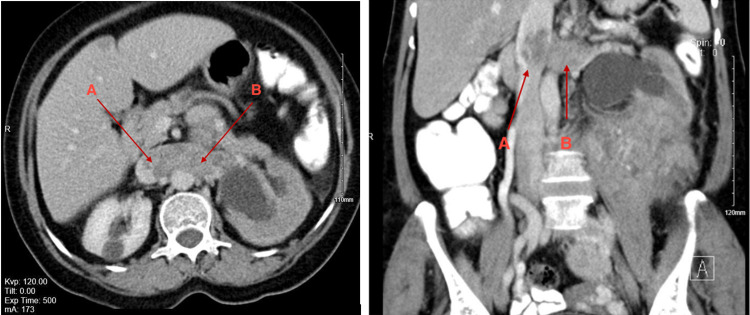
CT scan with contrast showed involvement of IVC and Left Renal Vein as pointed with the arrows. In both left-hand (axial view) and right-hand side (coronal) CT scan images- A: leiomyosarcoma invading inferior vena cava (IVC) B: leiomyosarcoma invading Left Renal Vein
